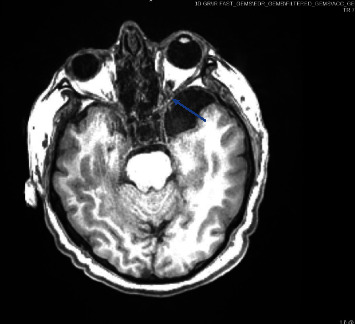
MRI of the brain of a 50-year old female with prominent optic nerve sheaths. Demonstrated by the blue arrow.
